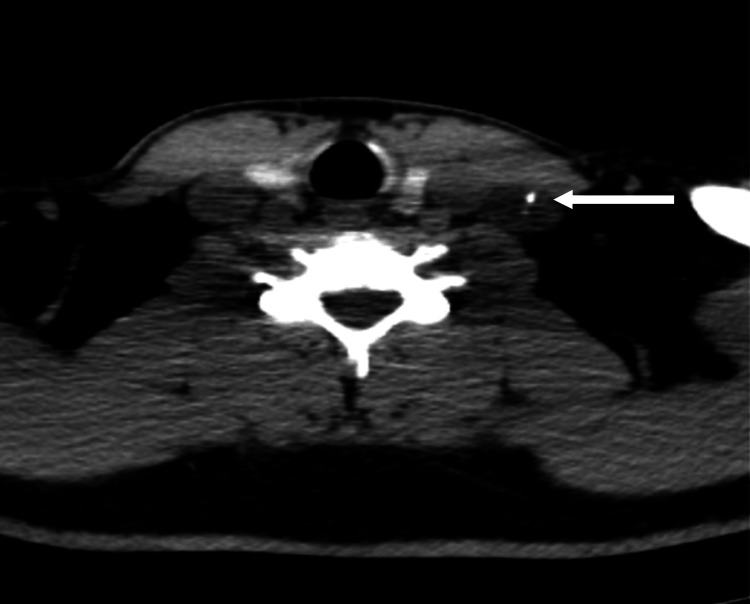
Neck imaging. Sestamibi scintigraphy showing a possible parathyroid adenoma measuring 1.5 x 0.7 x 0.4 cm in the left thyroid.
